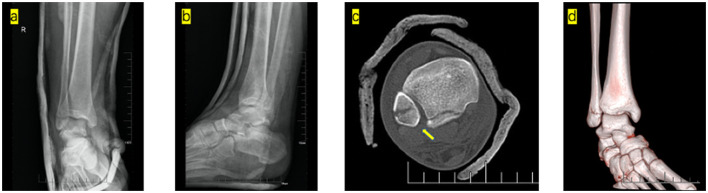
Post-manipulative radiographs show the anatomical alignment of the ankle mortise following closed reduction and external fixation. Anteroposterior (a) and lateral (b). Post-manipulative CT showed normal tibiofibular syndesmosis (yellow arrow). CT axial scans (c) and 3D CT (d).
